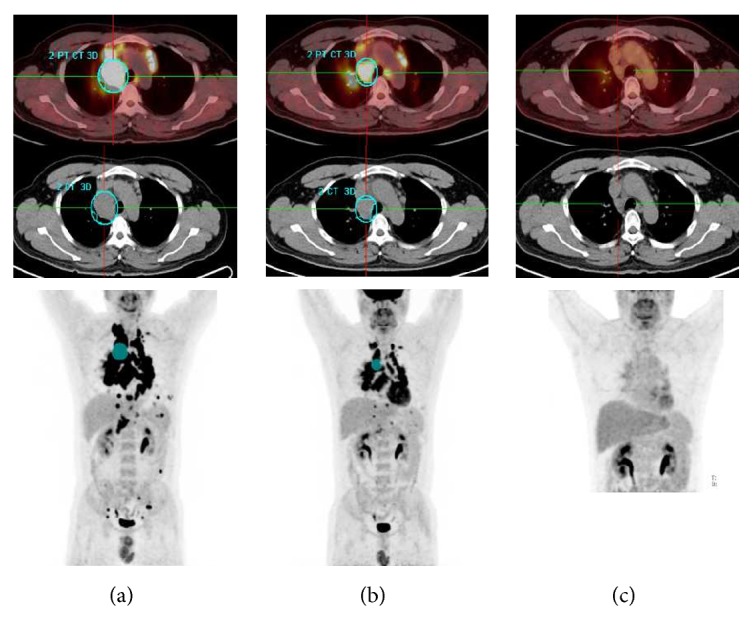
Results for patient 1, a 37-y-old man with symptomatic sarcoidosis. (a) A baseline ^18^F-FDG PET image revealed increased activity in mediastinal lymph nodes, lung parenchyma, liver, and spleen. (b) On the follow-up examination performed at 1 month, PET-CT showed significantly less activity in the mediastinal lymph nodes, liver, and spleen. However, his symptoms persisted but were less severe. (c) On the follow-up examination performed at 2 months, all abnormal uptakes were not apparent.
